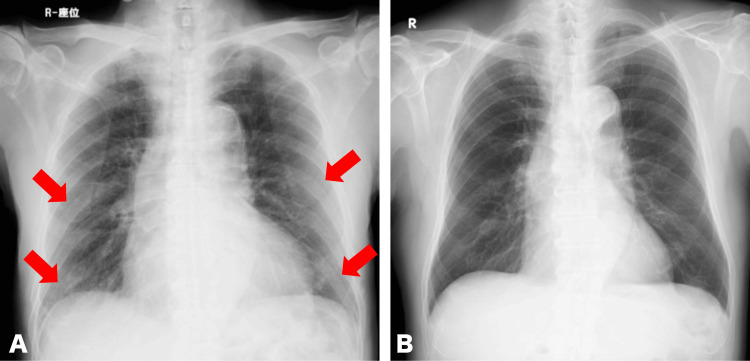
Chest X-ray (A) Ground glass opacity (arrow), at the first visit (seated position, AP view). (B) Disappeared abnormal shadows, at the follow-up visit (standing position, PA view).
